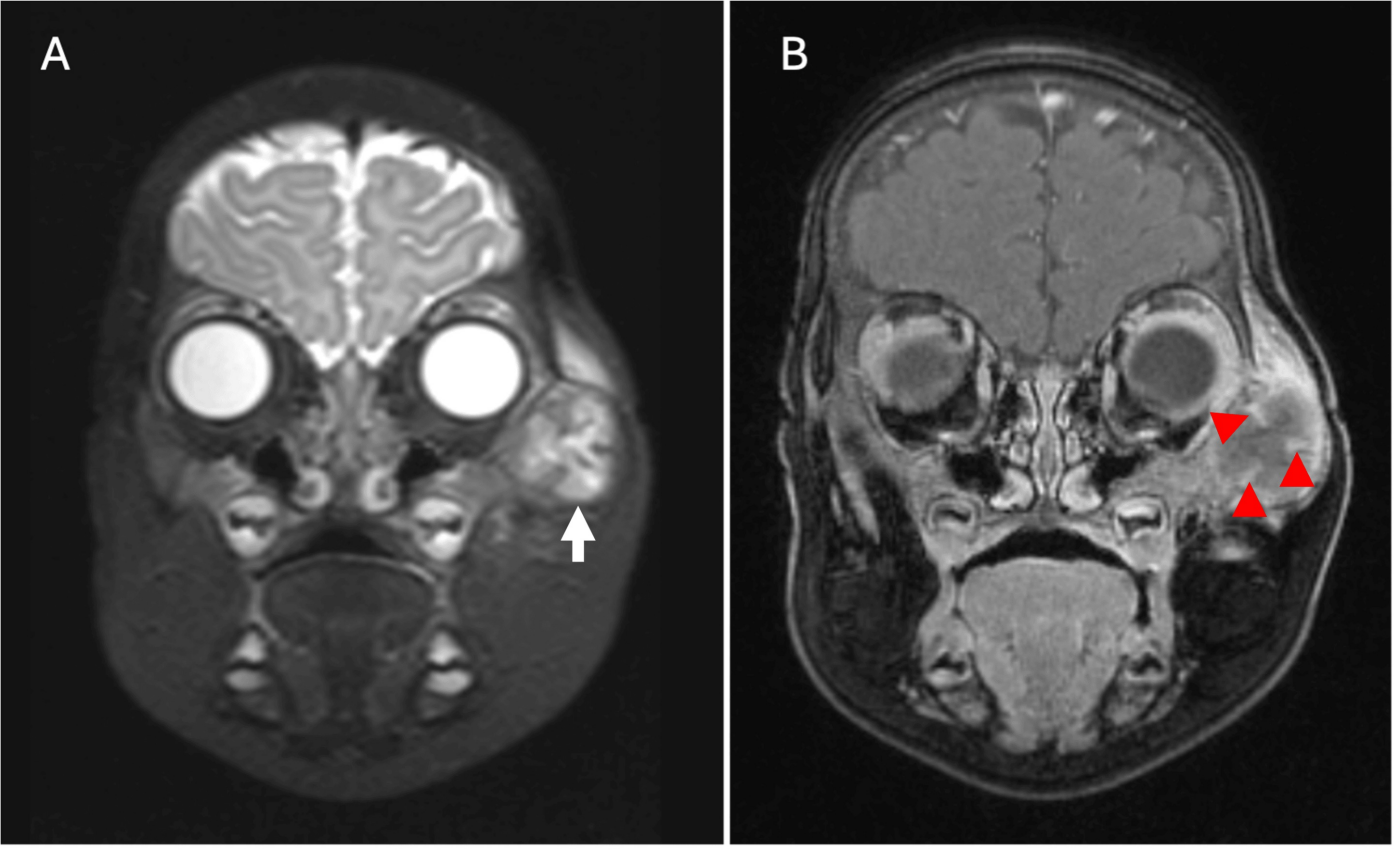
Coronal T2-weighted (A) and coronal T1-weighted post-contrast (B) images demonstrate a septated T2-hyperintense infraorbital lesion centered in the left zygomatic bone (arrow) with associated peripheral and septal enhancement (arrowheads).
